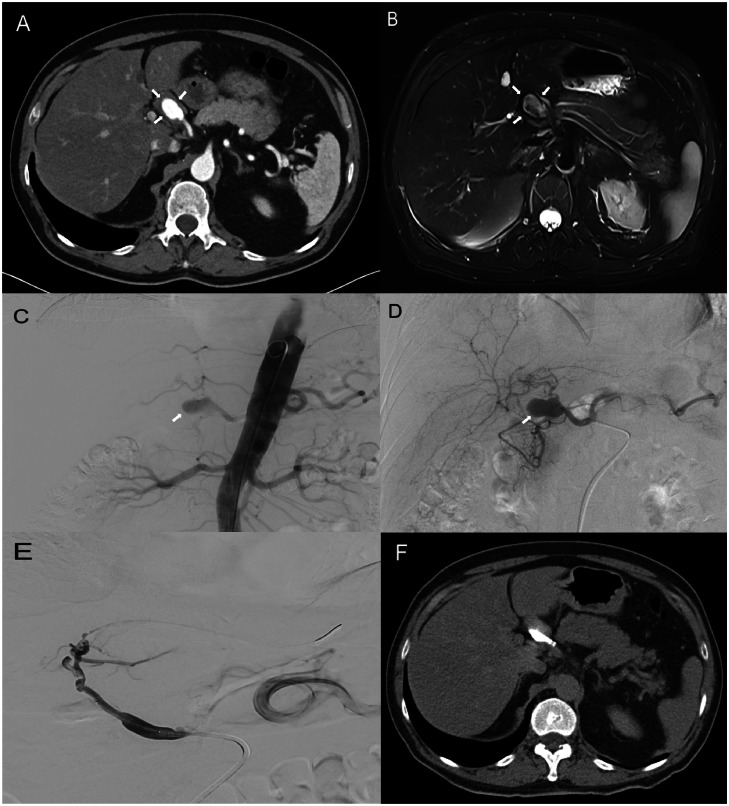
(A and B) Preinterventional contrast enhanced axial CT and axial T2-weighted fat-saturated MRI, show a saccular structure adjacent to the hepatic artery, representing the partially thrombosed saccular aneurysm (white arrows) of the proper hepatic artery with an approximate size of 2.6 × 3 cm. (C) Overview angiography using a pigtail catheter to demonstrate the branches of the abdominal aorta. (D) Selective catheterization of the hepatic artery, hereby the saccular aneurysm bordering the hepatic artery can be seen (white arrow). (E) Shows the vascular state after stent implantation, wherein the aneurysm is no longer perfused. (F) Postinterventional axial CT-scan did not show any complications.
